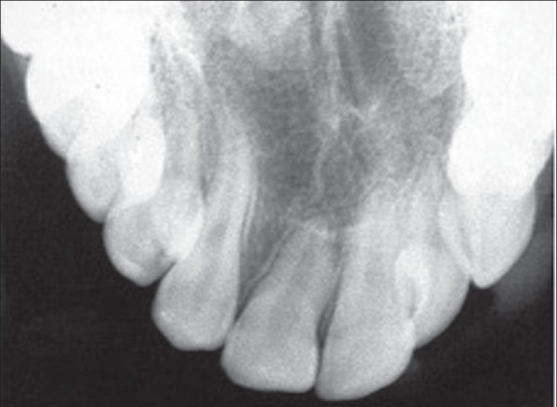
Post Alveolar Bone Graft Xray
